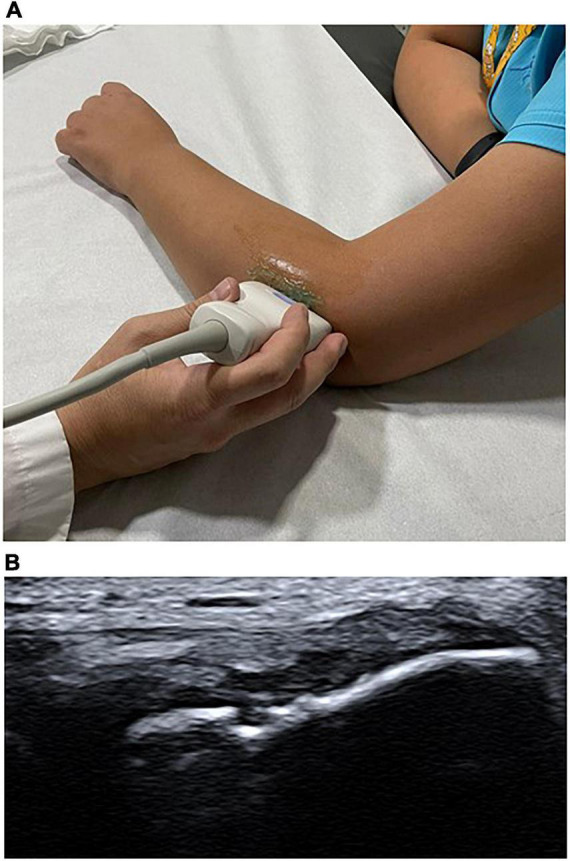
Patient evaluation position and an ultrasound (US) finding, respectively. (A) Probe positioning in the elbow in the US exploration of the extensor tendon complex. (B) US imaging shows intrasubstance tear in extensor tendon complex.
